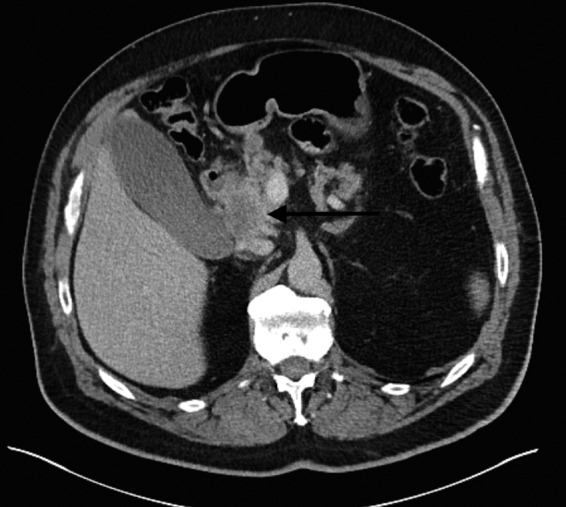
Axial contrast-enhanced computed tomography (CT) image demonstrates a defined hypo-attenuating mass in the head of the pancreas (*arrow*), which abuts the second portion of the duodenum.
